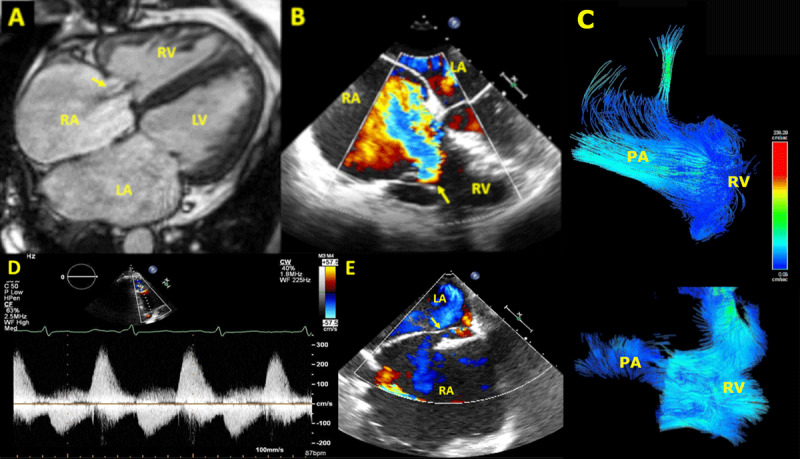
Severe PV regurgitation leading to severe functional TV regurgitation, favoring a right-to-left shunt via PFO. Severe TV regurgitation jet shown by 4-chamber view (A) CMR and (B) transesophageal echocardiography. (C) Four-dimensional CMR demonstrating severe PV regurgitation. (D) Spectral doppler showing classic PV regurgitation waveform. (E) PFO with right-to-left shunt by transesophageal echocardiography. LA: left atrium; LV: left ventricle; PA: pulmonary artery; PFO: patent foramen ovale; PV: pulmonary valve; RA: right atrium; RV: right ventricle; TV: tricuspid valve.
